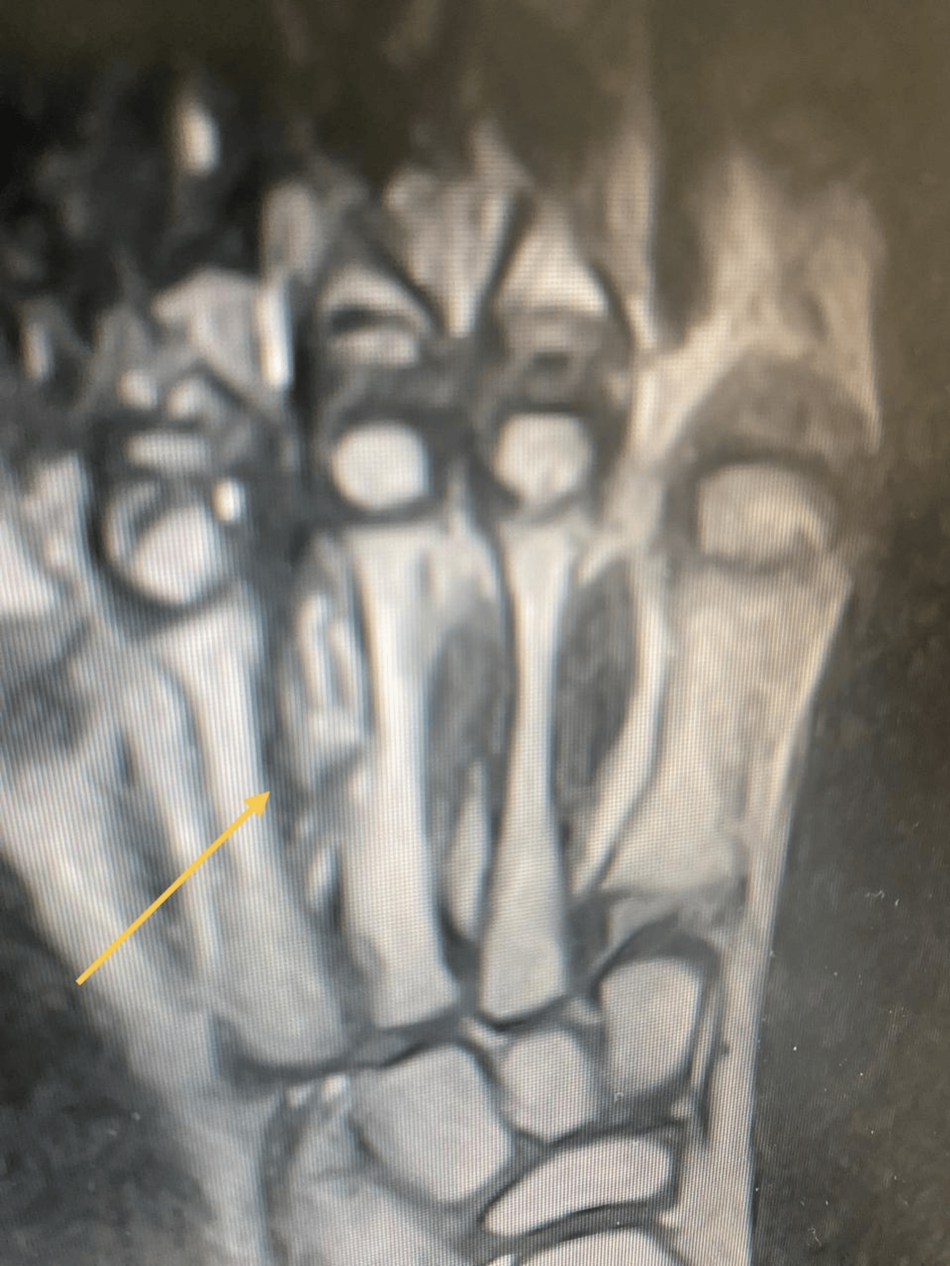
Rt foot MRI. Multi-planner MRI for the right foot with coronal PD FS, sagittal T1, PD FS, axial T2 and PD FS was performed. Limited study with poor quality at the third metatarsal bone; the mid portion shows evident cortical thickening and mild soft tissue edema. PD FS: Proton density fat saturation
